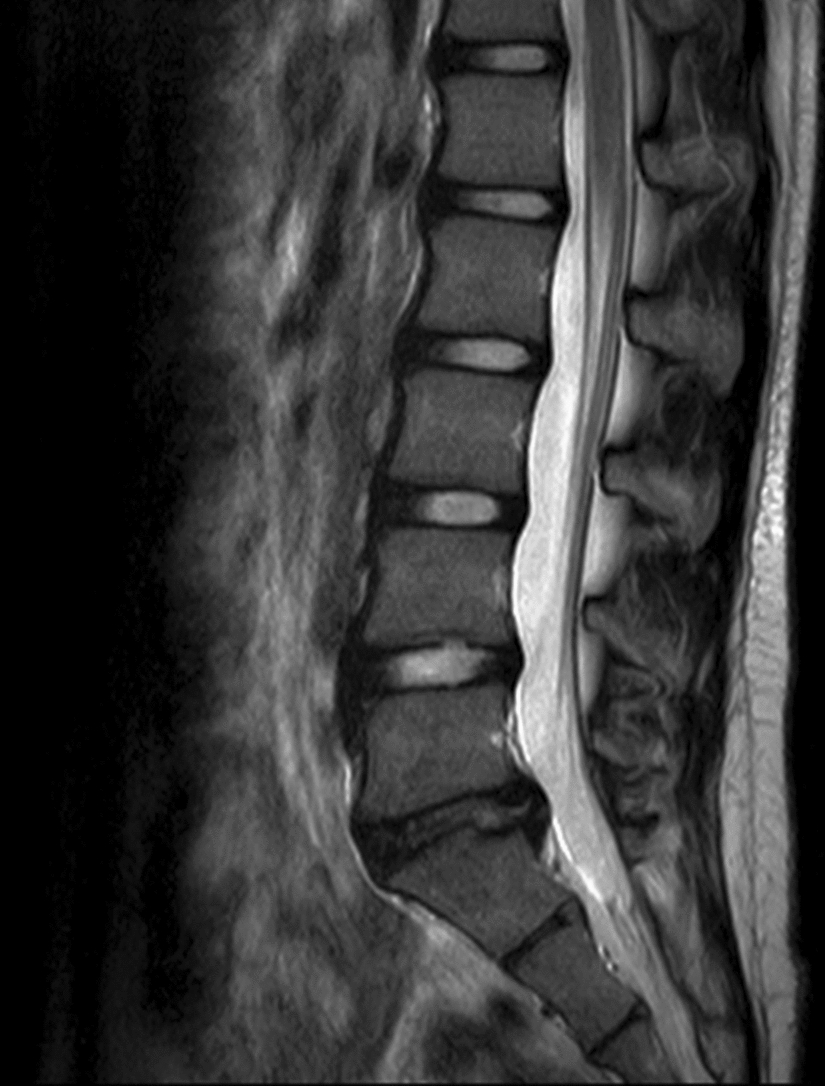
MRI obtained at the girl’s 30-month follow-up showed no significant changes in Schmorl’s node at L4 and S1, platyspondyly of the lumbar vertebrae, and no significant resorption of the herniated L5/S1 lumbar disc
